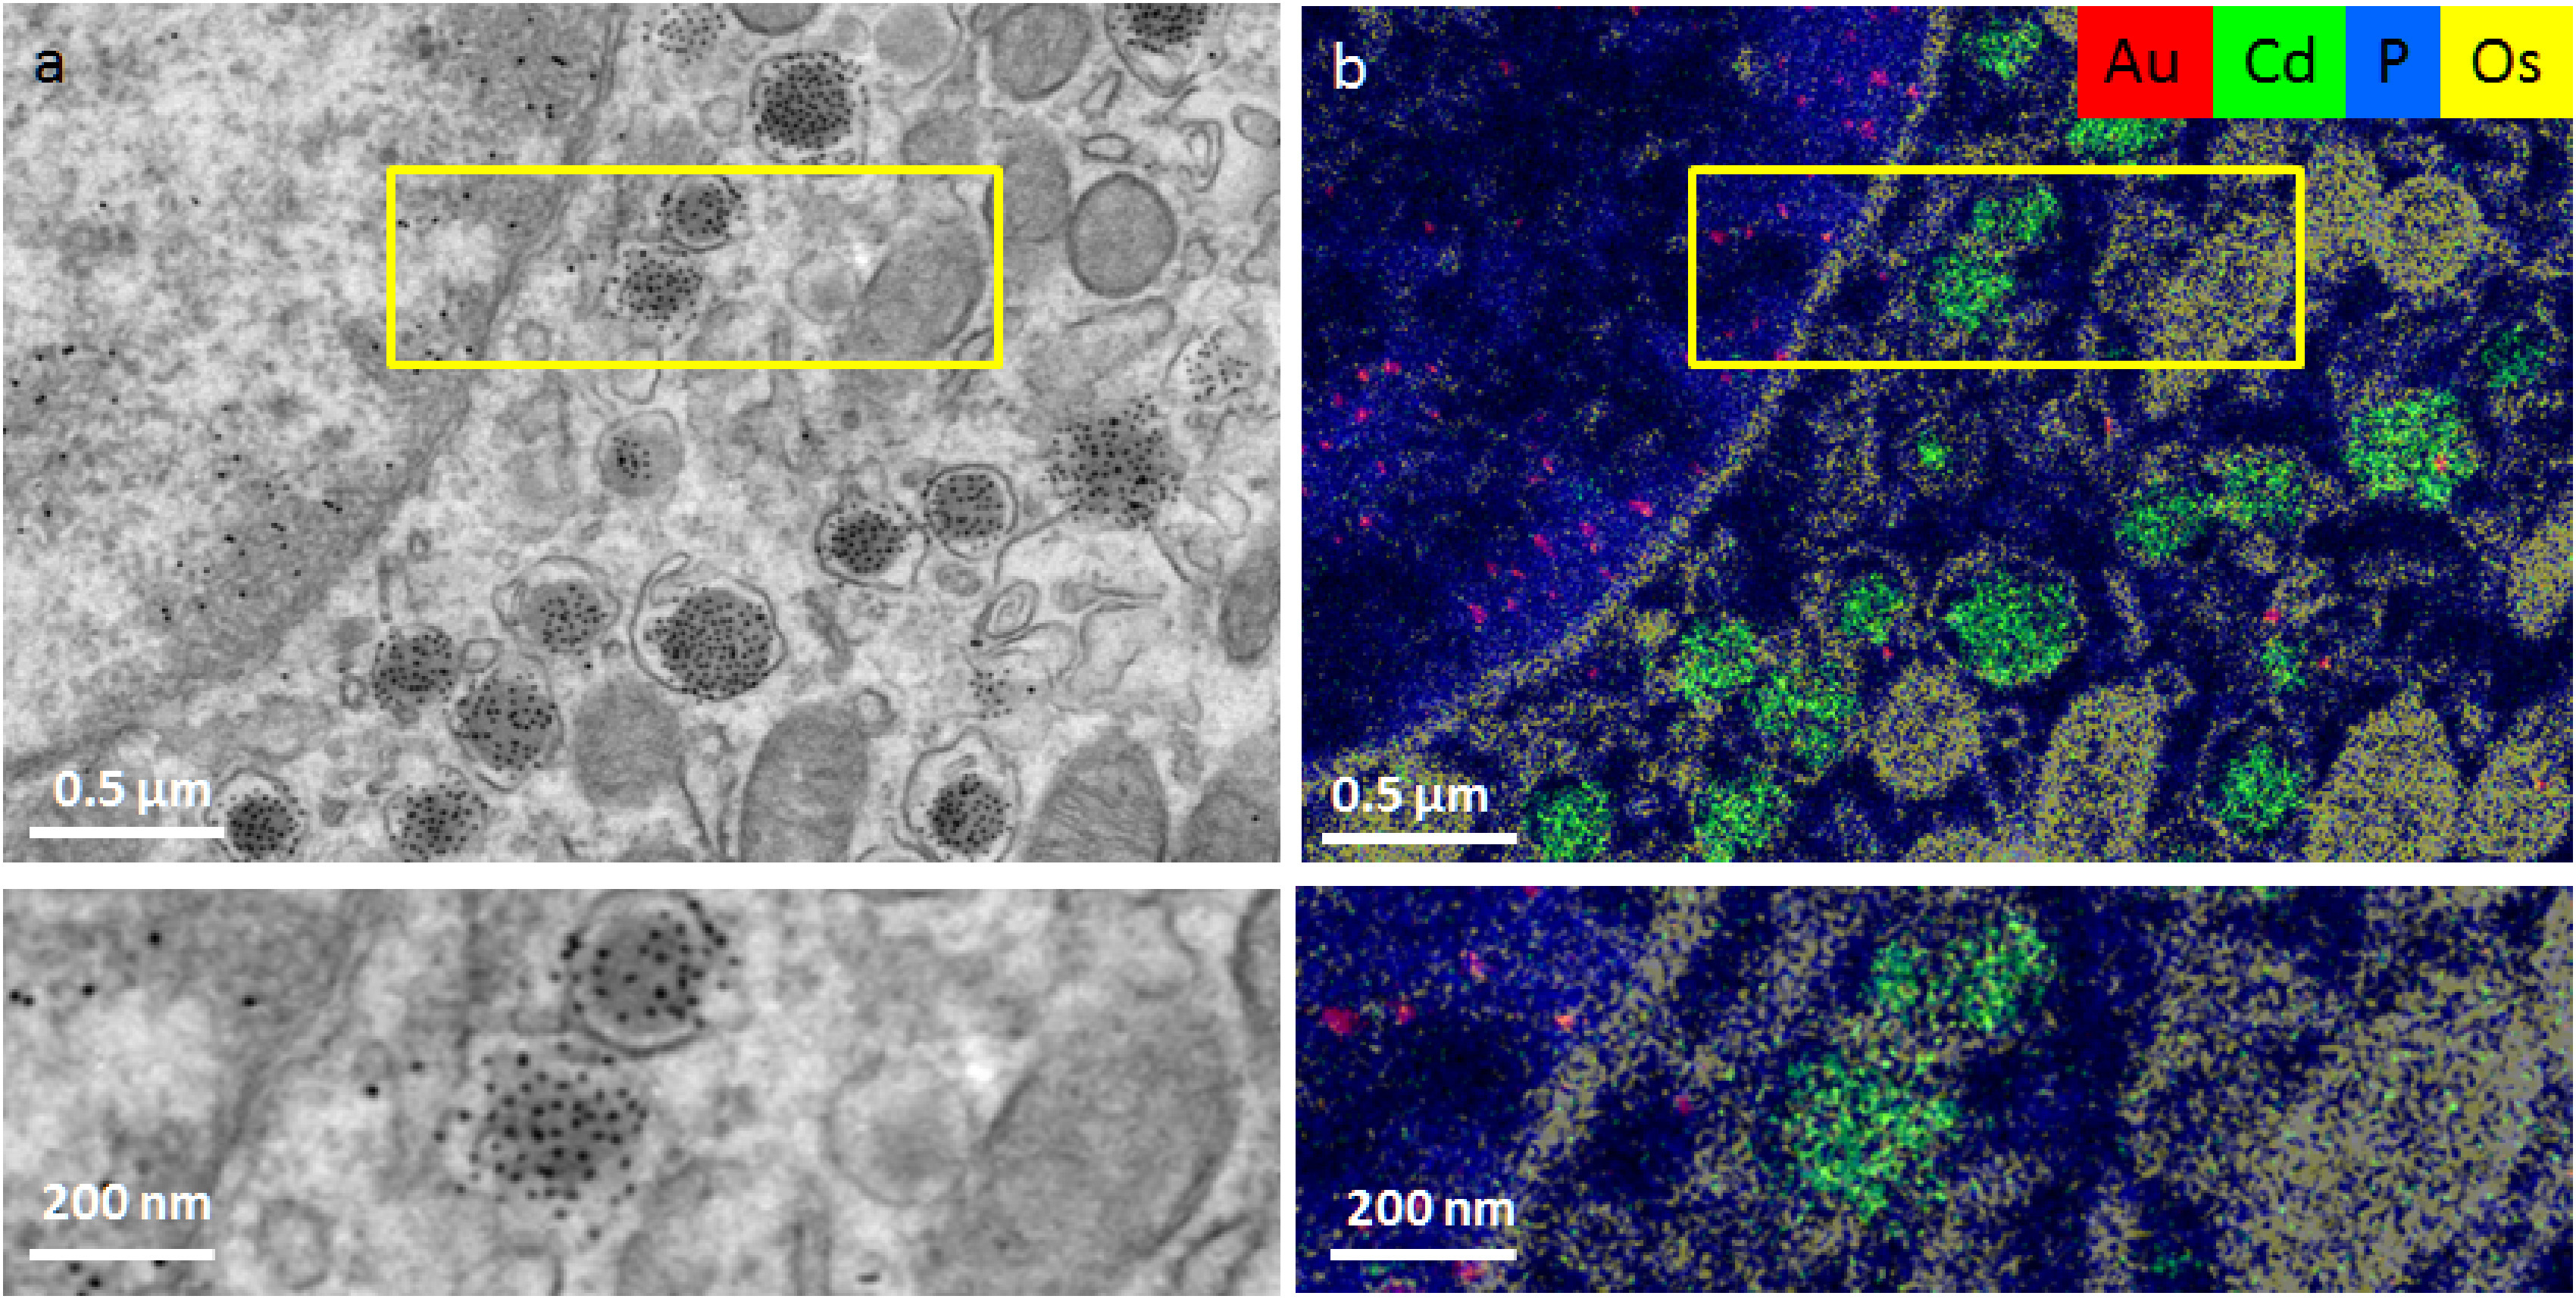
‘ColorEM’ using elemental analysis by energy dispersive x-ray imaging. ColorEM: label-free (P), paint (Os) and labeling DNA (Au) and peptides (Cd) is compatible. (a) Part of an islet of Langerhans immuno-labeled for structures in DNA (10 nm gold) and insulin (QD). (b) Overlay image of Au (red), Cd (green), Os (yellow) and P (blue) allows identification of G4 structures (gold labels) and insulin (Cd). Note the localization of Au to heterochromatin enriched in P, whereas the Cd signal is enclosed within a combination of Os rings and P that likely identifies phospholipid membranes of the vesicles. Large scale data and full resolution data is available via www.nanotomy.org; Reproduced from [22]. CC BY 4.0.
